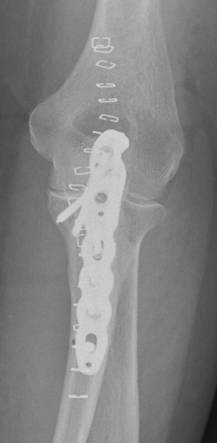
Postoperative biplanar X-rays show situation after internal fixation.
